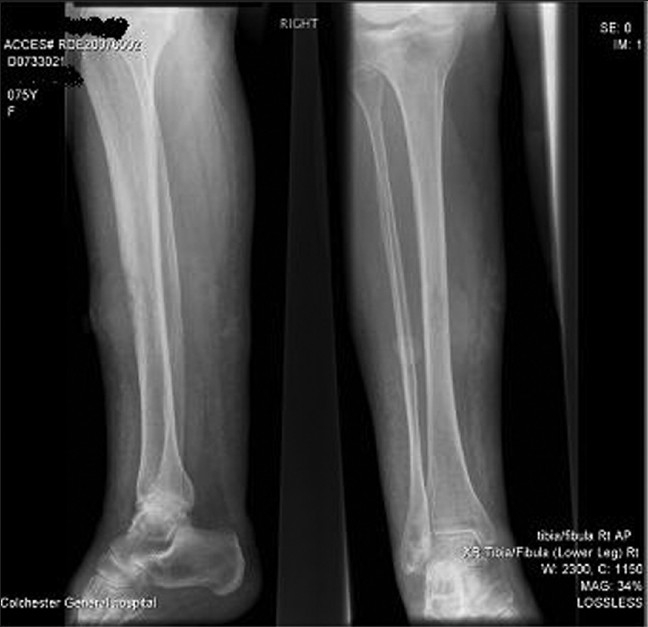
Plain radiograph of right tibia and fibula showing cortical erosion of lower third of tibia
